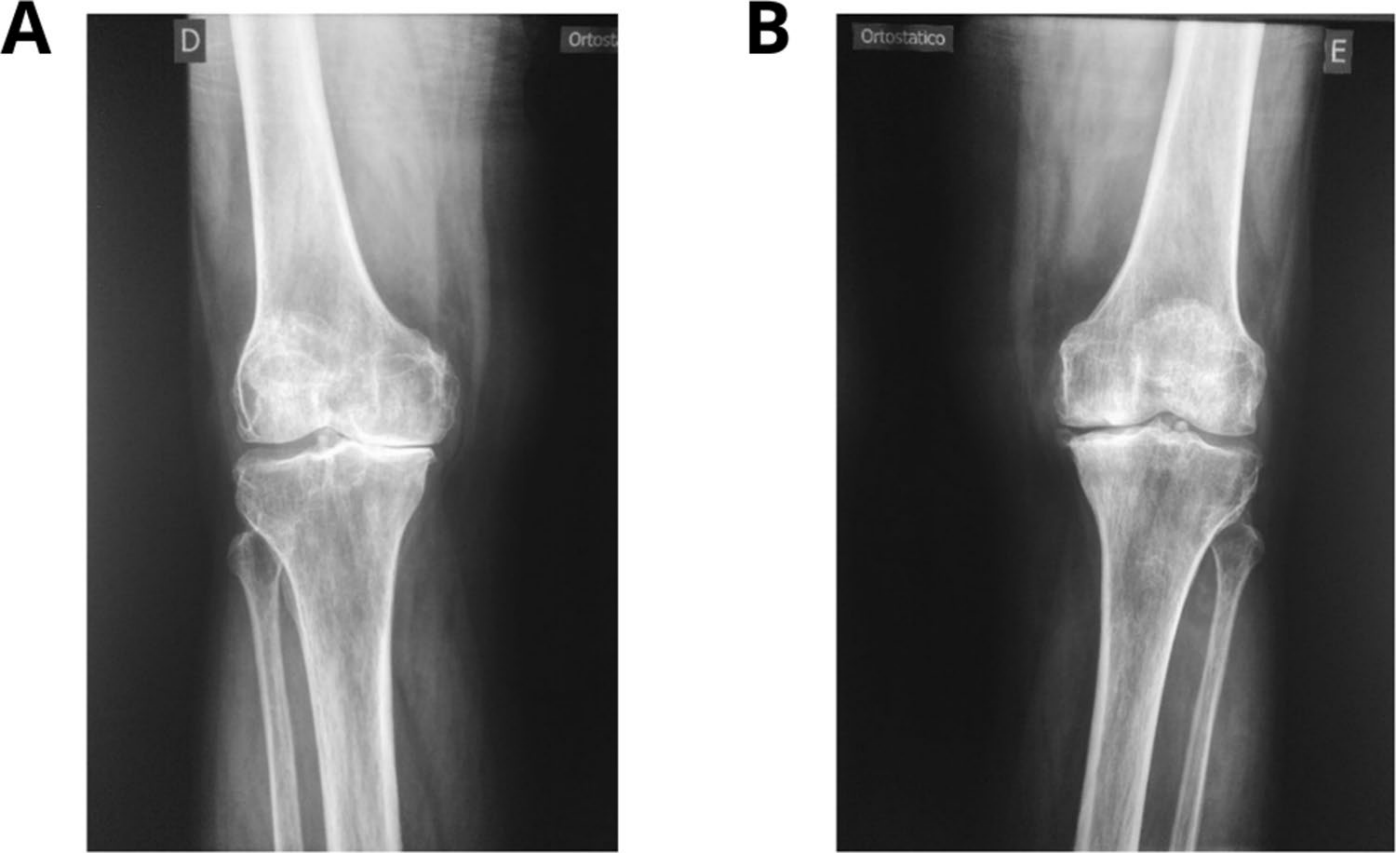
Knee X-ray. (A) Right knee, osteoarthritis Kellgren & Lawrence grade IV. (B) Left knee, osteoarthritis Kellgren & Lawrence grade III.
